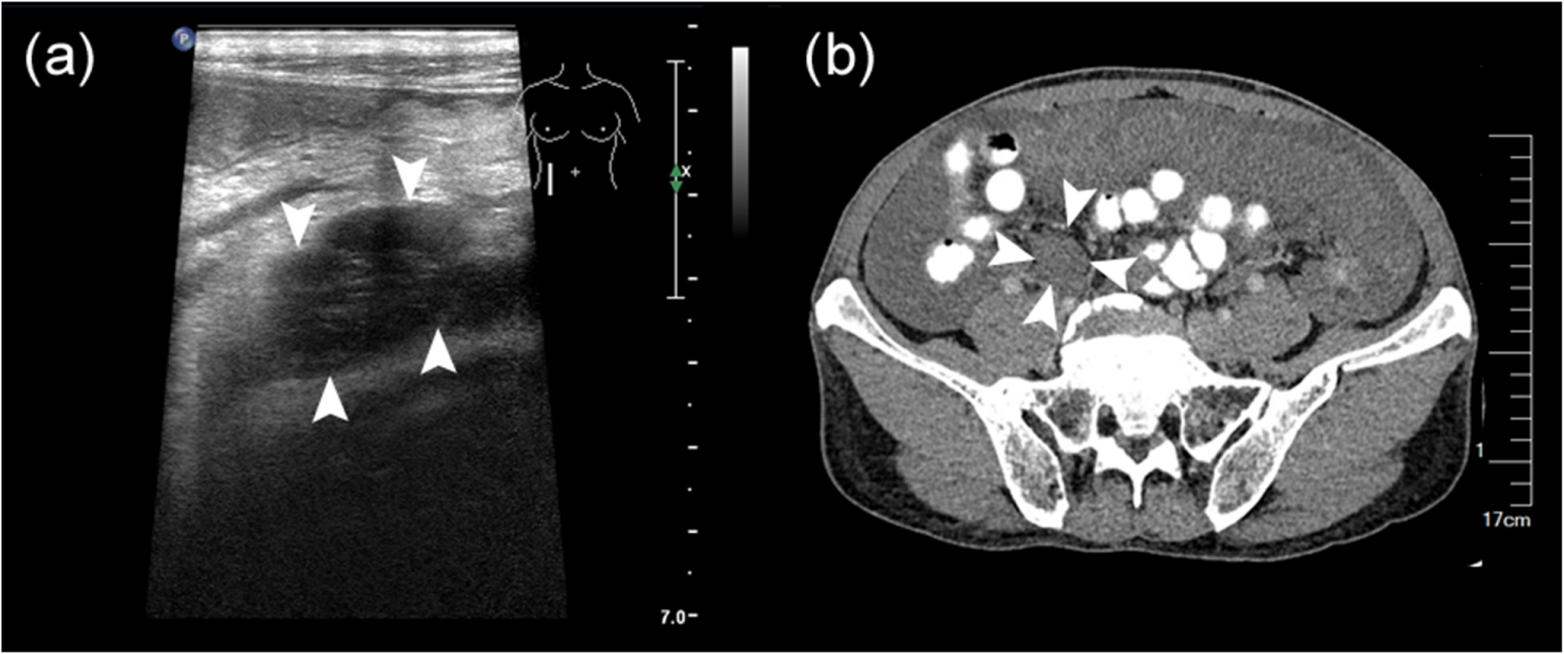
The typical imaging features in US and contrast CT. a and b showed mesenteric involvement in US and CT
